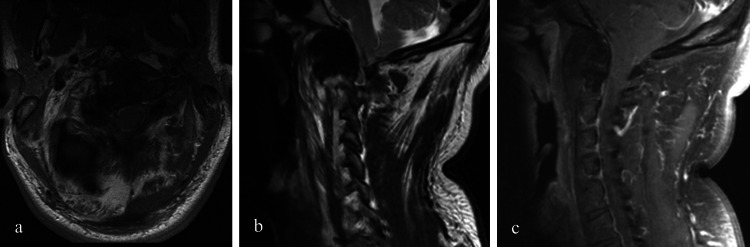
MRI of the cervical spine with axial T1-WI (a), sagittal T2-WI (b) and fat supressed contrast-enhanced sagittal T1-WI (c). Besides diffuse lipomatous infiltration, axial T1-WI (a) and sagittal T2-WI (b) show a hypointense mass at the atlanto-axial joint, extending into the anterior epidural space, but without compression of the brainstem or the spinal cord. No contrast enhancement is depicted on fat-suppressed contrast-enhanced sagittal T1-WI (c).
